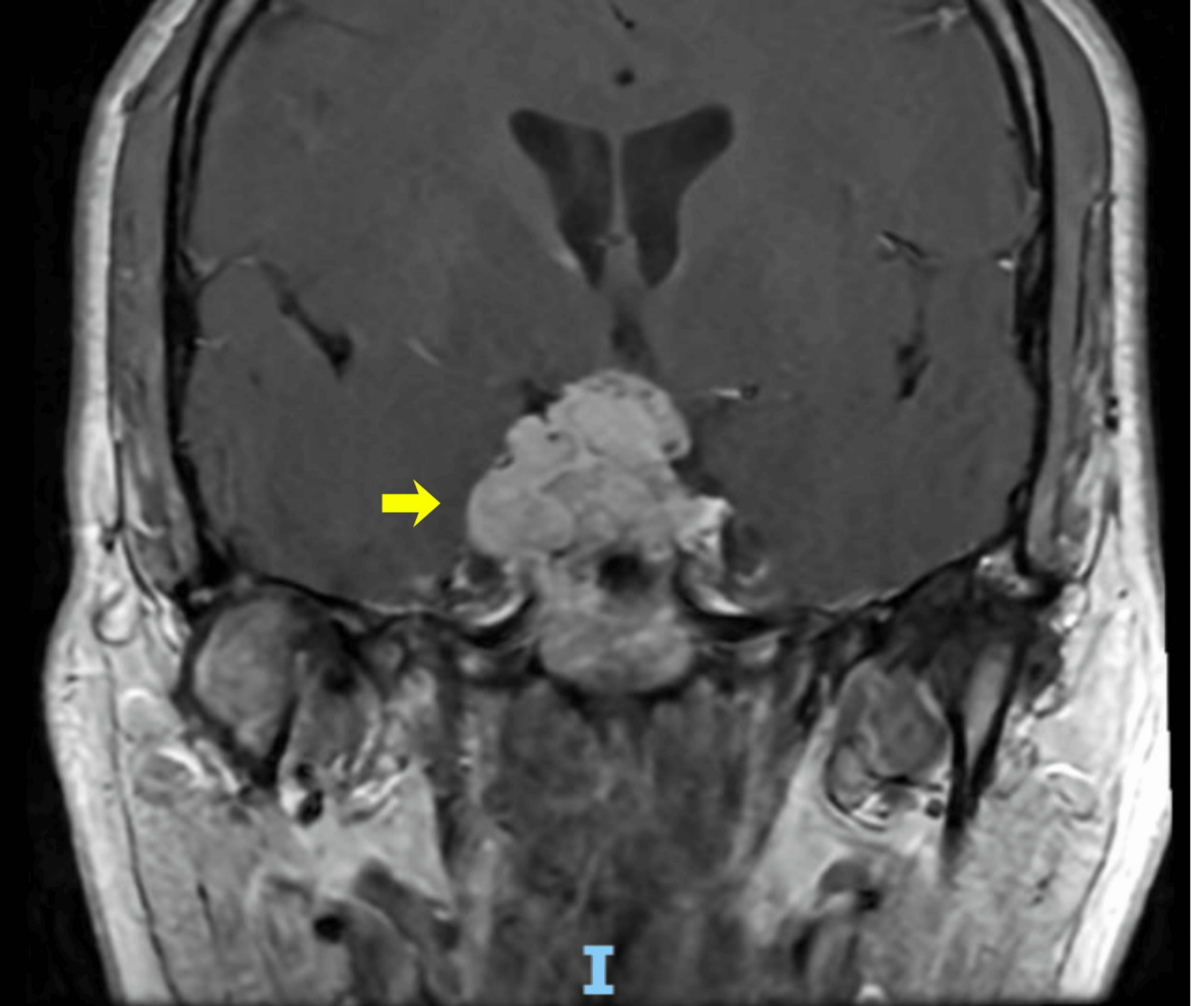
Coronal contrast-enhanced magnetic resonance imaging Coronal contrast-enhanced magnetic resonance imaging demonstrating a pituitary mass with heterogeneous enhancement and suprasellar extension, with the compression of the optic chiasm (yellow arrow)
